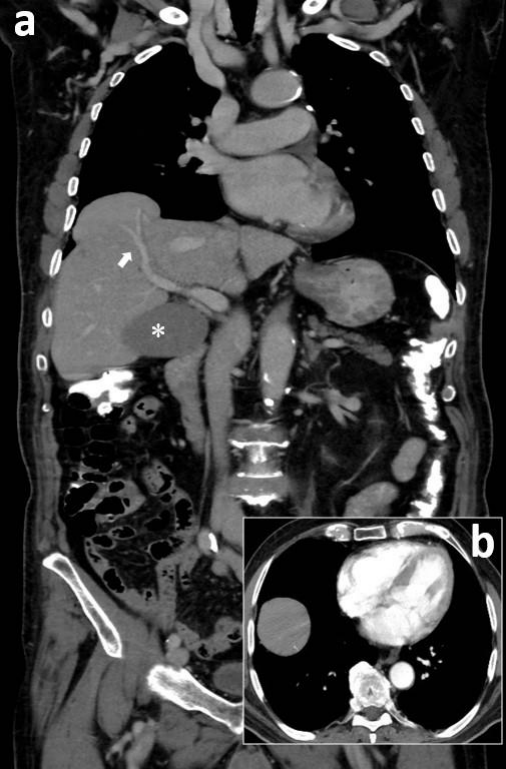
contrast enhanced computed tomography; a) multiplanar reconstruction showing the supradiaphragmatic ectopic liver and its vascularity (arrow); star: echinococcal cyst; b) axial view: showing a mildly enhancing well circumscribed, soft tissue mass in the right hemithorax
